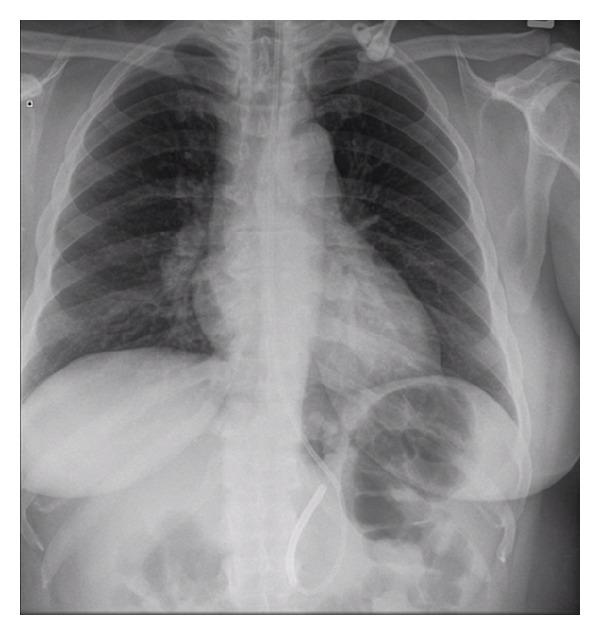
Chest X-ray showing hilar lymphadenopathy. Also of note is a nasogastric tube that was required for nutrition due to difficulty with swallowing.
